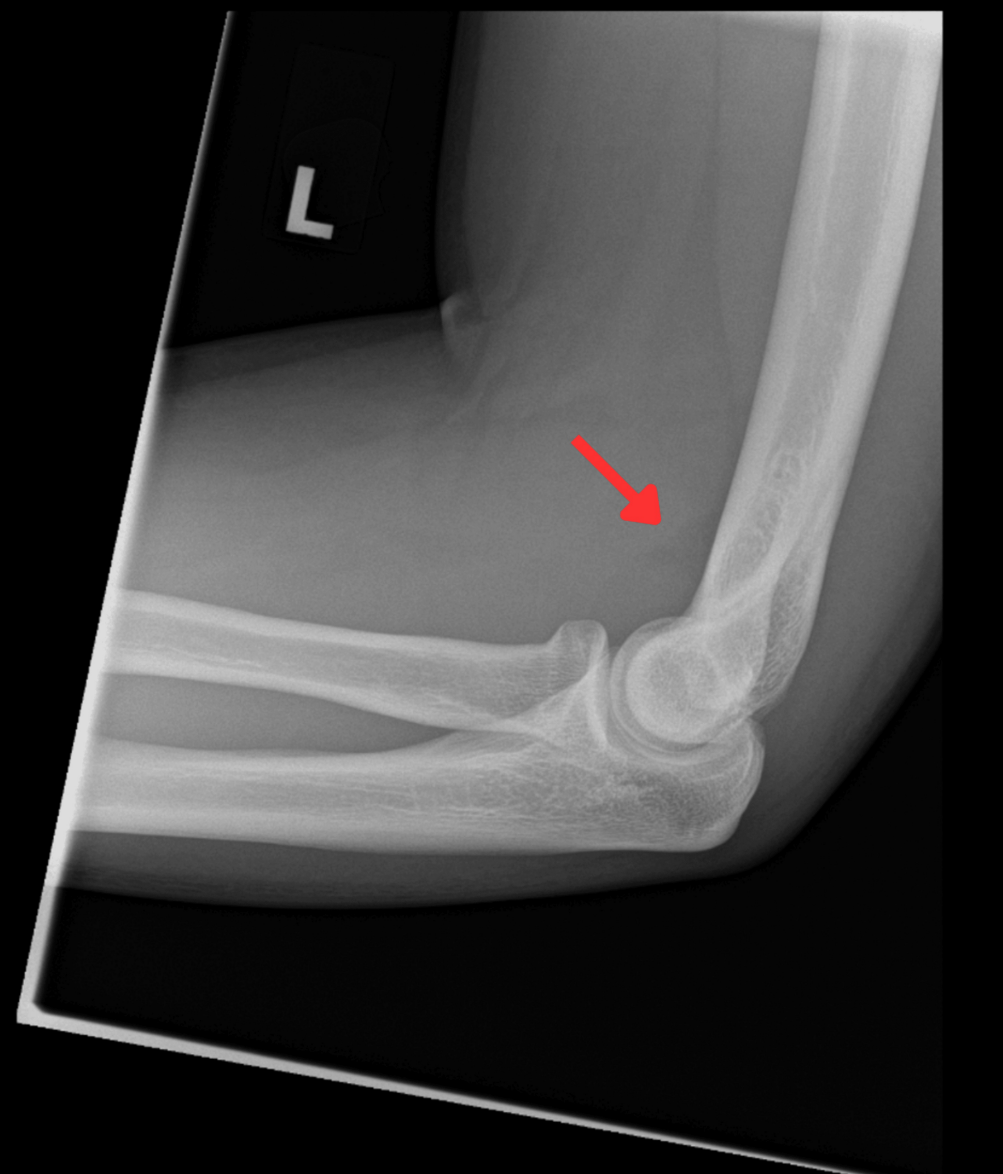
X-ray lateral view of the patient's left elbow Red arrow: Raised anterior fat pad indicating effusion of the left elbow
